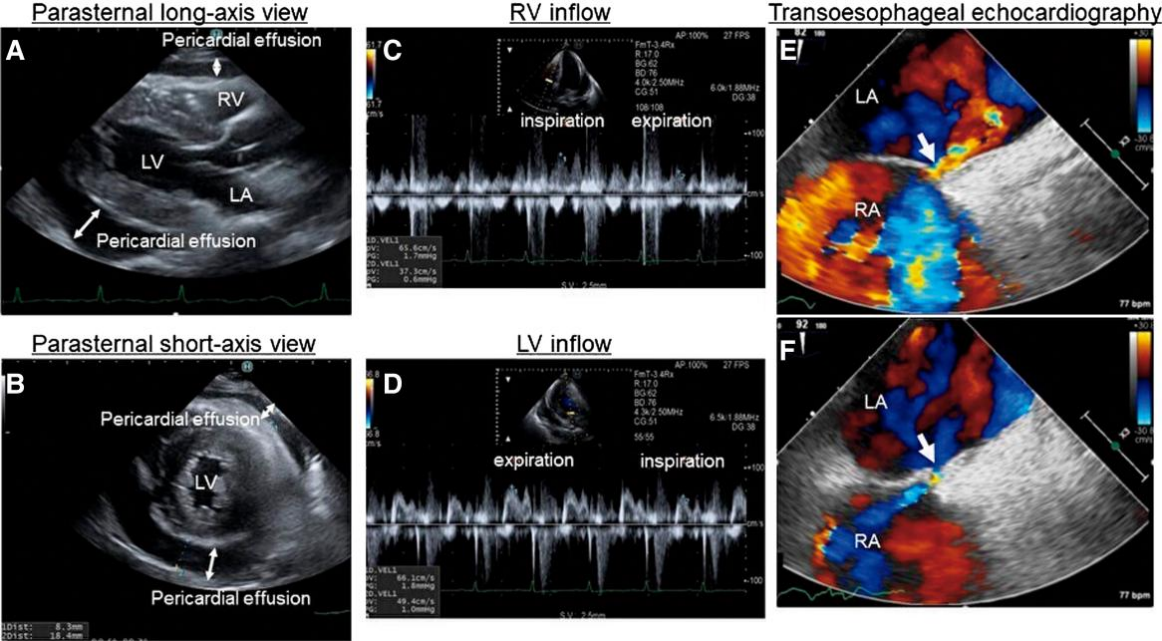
Transthoracic echocardiography images. Transthoracic echocardiography (parasternal long-axis and short-axis views) shows a large circumferential pericardial effusion (*A* and *B*). A pulsed-wave Doppler echocardiogram shows a 25% decrease in the E-wave velocity of the left ventricular inflow during inspiration, while the E-wave velocity of the right ventricular inflow increased by 76% during inspiration (*C* and *D*). Transoesophageal echocardiography shows no evidence of infective endocarditis and bidirectional shunt flow through patent foramen ovale (*E* and *F*). LA, left atrium; LV, left ventricular; RV, right ventricular.
